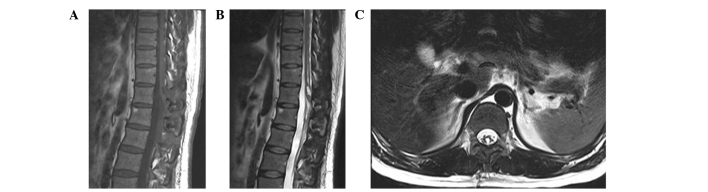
Contrast-enhanced (A) T1-weighted, (B) T2-weighted and (C) coronary T2-weighted magentic resonance images at nine months postsurgery showing no recurrence of the schwannoma or the cyst, and indicating the cavity left by the removal of the mass in the spinal cord.
